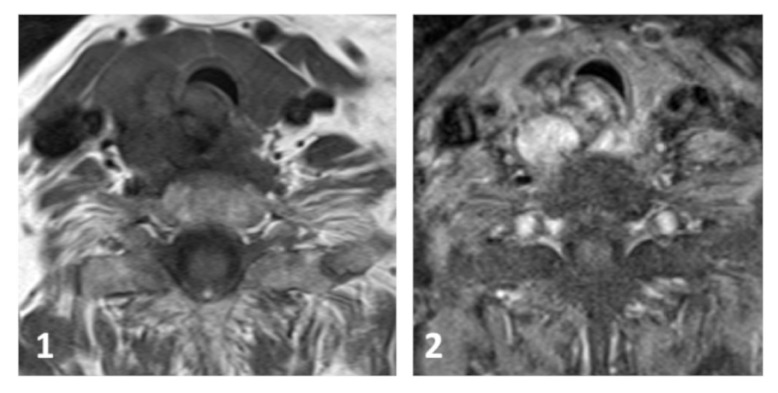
Axial T1-weighted MR image (1) and postcontrast image (2) show a large mass near the right trachea without infiltration.
